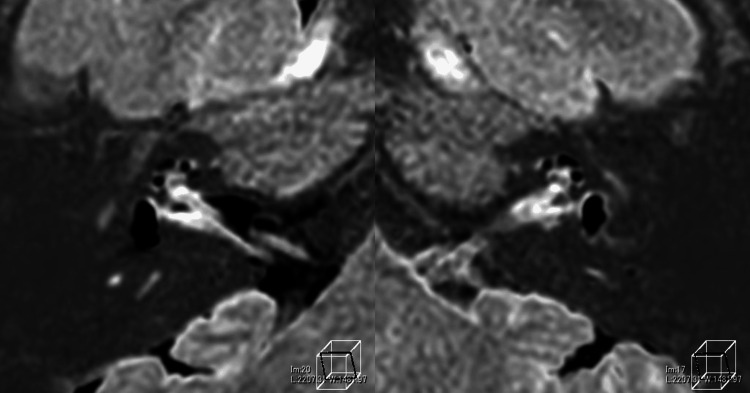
Pre-operation MRI (HYDROPS). Marked endolymphatic hydrops in the bilateral cochlea and vestibule were observed.
